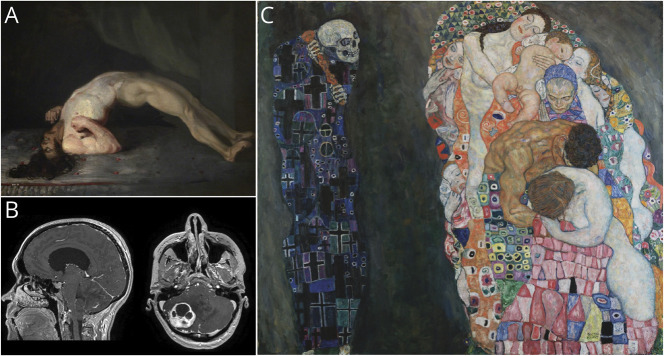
Examples of Clinical and Art Imagery Used in the Preintervention and Postintervention Tests (A) Charles Bell, The Wounded following the Battle of Corunna: Tetanus Following Gunshot Wounds, Photo © The Royal College of Surgeons of Edinburgh. Reprinted with permission from the Royal College of Surgeons of Edinburgh. (B) Sagittal and axial T1-weighted, postgadolinium images from a brain MRI demonstrating a hemangioblastoma of the right cerebellar hemisphere with associated mass effect and tonsillar herniation. (C) Gustav Klimt, Death and life, Photo © Leopold Museum, Vienna. Reprinted with permission from the Leopold Museum.
